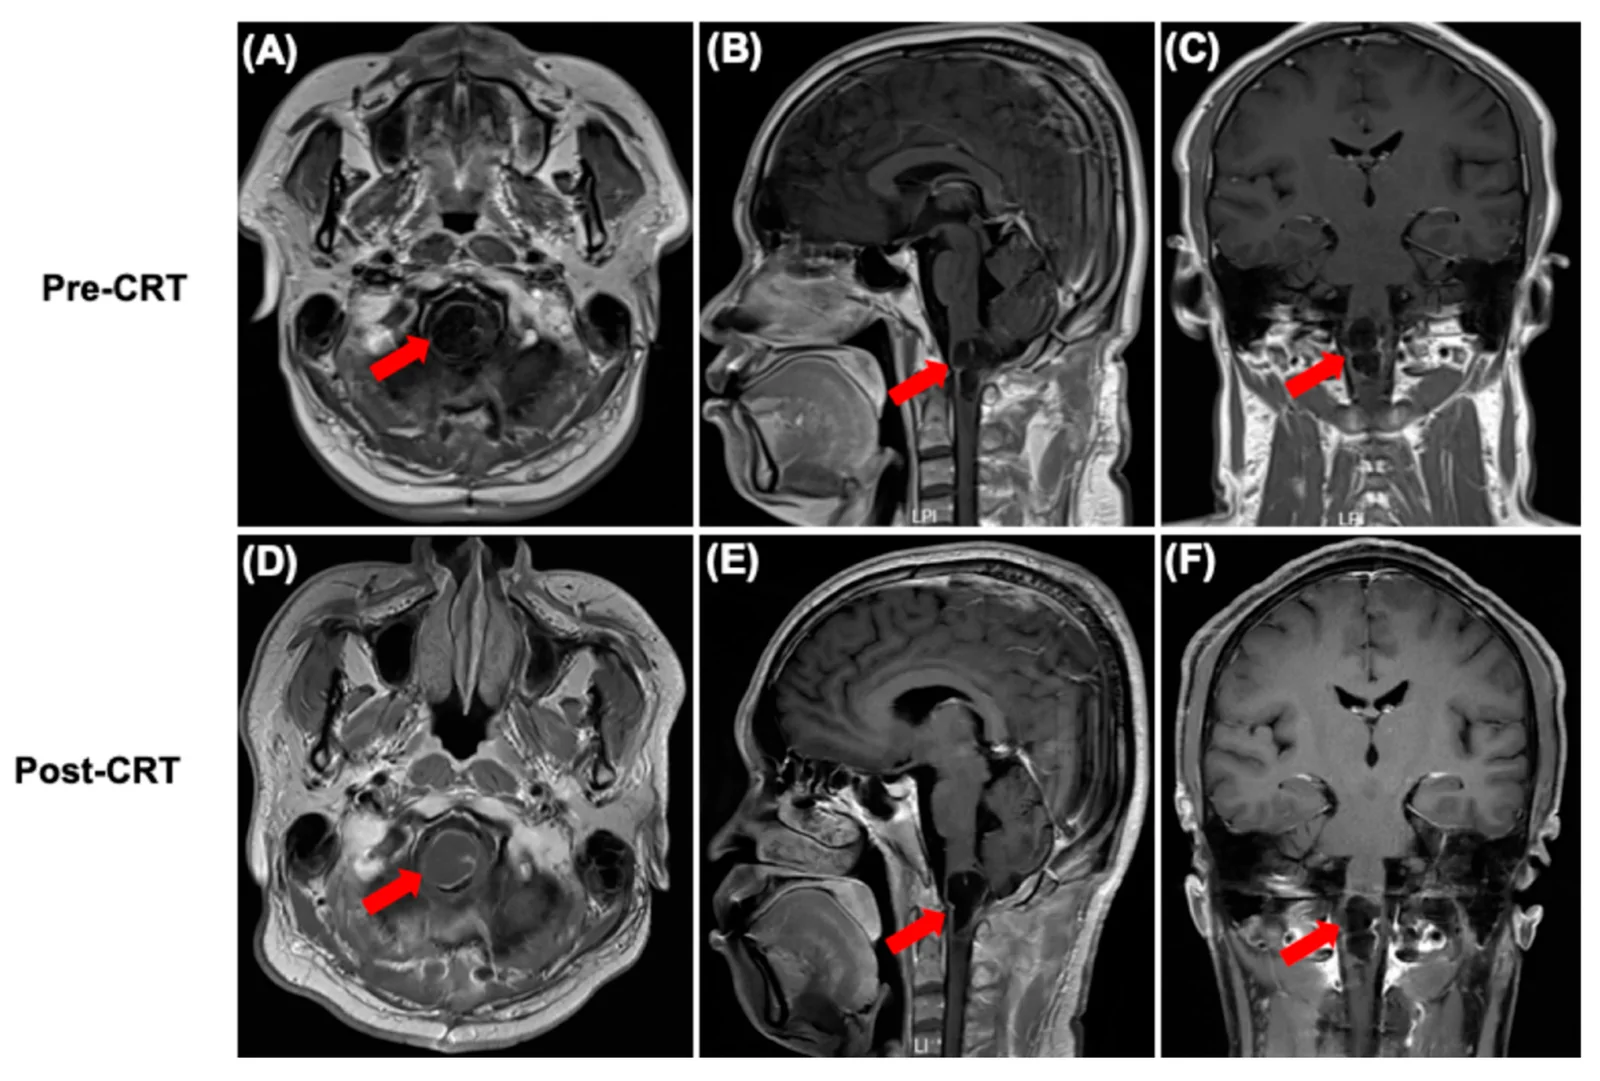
Cranial MRI showing brainstem lesions before and after CRT. (A–C) Axial, sagittal, and coronal views before CRT; (D–F) Axial, sagittal, and coronal views after CRT. The position pointed by the arrow is the site of the lesion.
